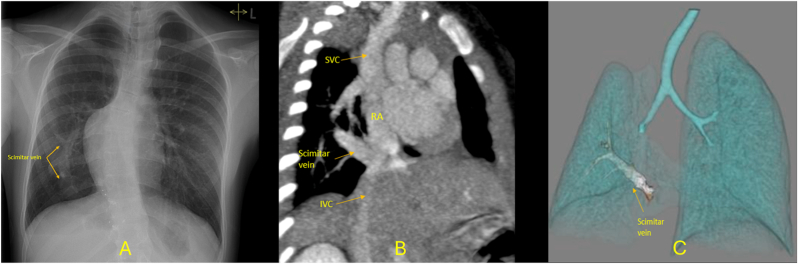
(A) Chest X-ray from a 15-year-old girl shows a scimitar vein parallel to the right heart border in the shape of Turkish sword (Scimitar). (B, C) Images of a 6-month-old girl with SS with abnormal bronchial branching with a hypoplastic single lobed right lung: (B) Oblique CT reconstruction shows the scimitar vein from the right lung draining into the IVC at the inferior cavo-atrial junction; (C) 3D volume and surface rendered image shows abnormal bronchial branching with a hypoplastic single lobed right lung and the scimitar vein. SS: Scimitar Syndrome, CT: Computed Tomography, IVC: Inferior Vena Cava, 3D: Three-Dimensional.
